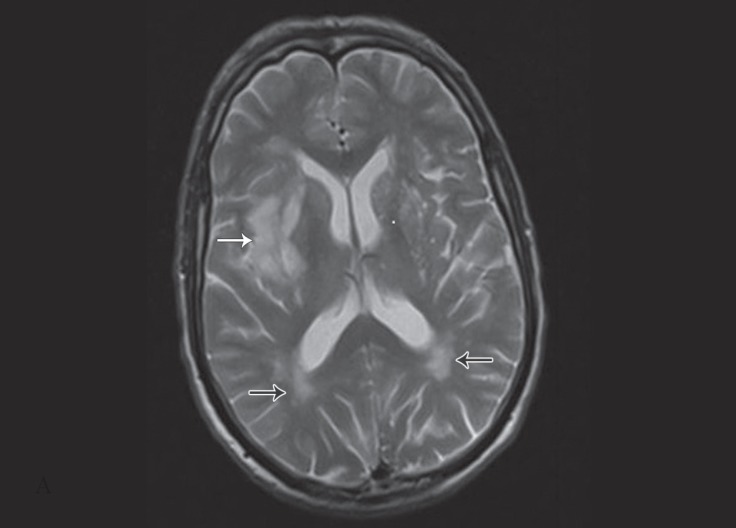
Brain magnetic resonance imaging without contrast shows an abnormal bright signal in the periventricular white matter in both cerebral hemispheres, in favor of microvascular ischemic changes (black arrows). Also, acute infarction can be seen in the right insula, external capsule, putamen, and caudate nucleuses (arrow).
